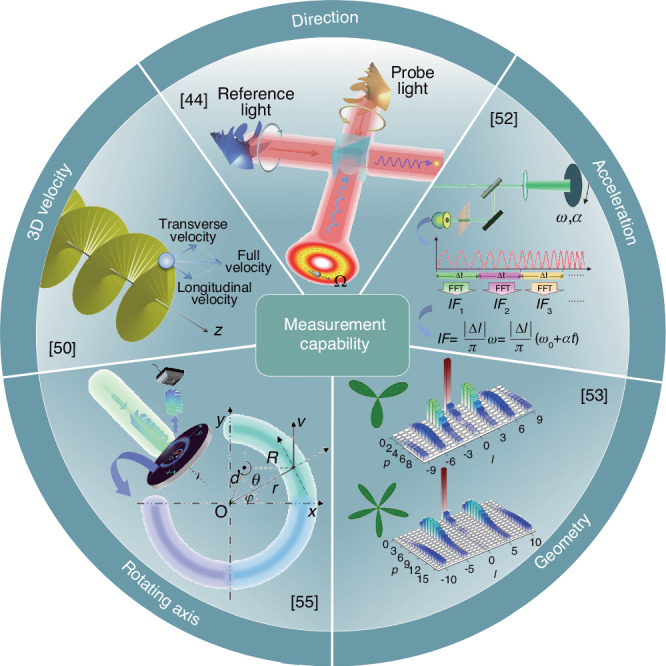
Advances in Doppler metrology. Doppler metrology technology has evolved from assessing the 2D rotational velocity to covering 3D velocity assessment, determination of rotational direction and acceleration, geometric symmetry analysis, and detection of axis orientation. 3D velocity trajectories of particles can be analyzed by revealing both translational and rotational velocities, including helical motions. The rotational axis and direction can be determined by using dynamic structured light, dual-frequency vortex beam, or vector RDE with oppositely polarized vortex beams. Time-frequency analysis dynamically tracks rotational velocity and obtains acceleration. RDE measurements exhibit high sensitivity to geometric symmetry and rotation axes of objects, enabling analysis of geometric symmetry and determination of rotation axes. References given in the insets
